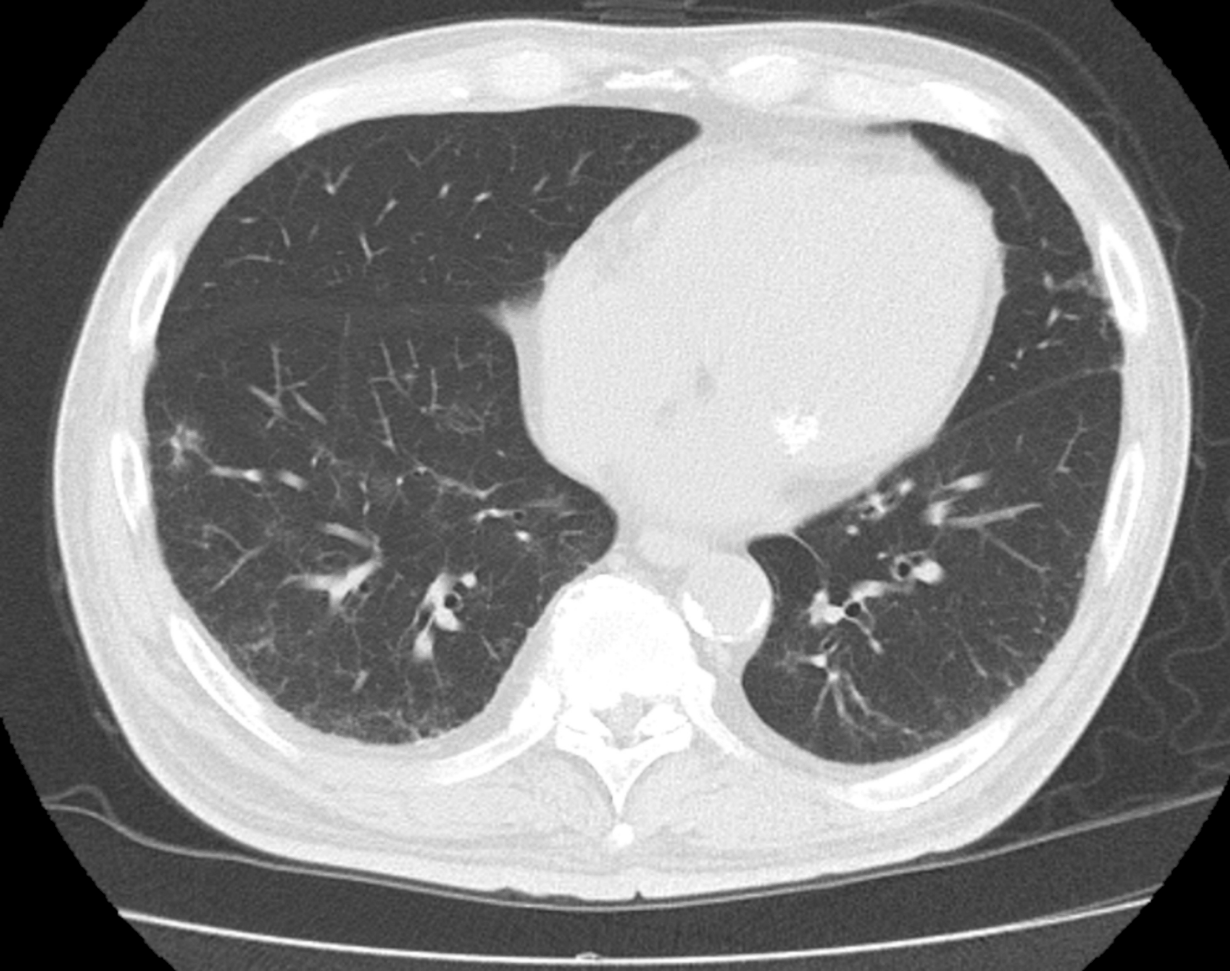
Post-treatment chest CT scan Ground-glass opacities show improvement. Bilateral pleural effusions persist. CT: computed tomography
